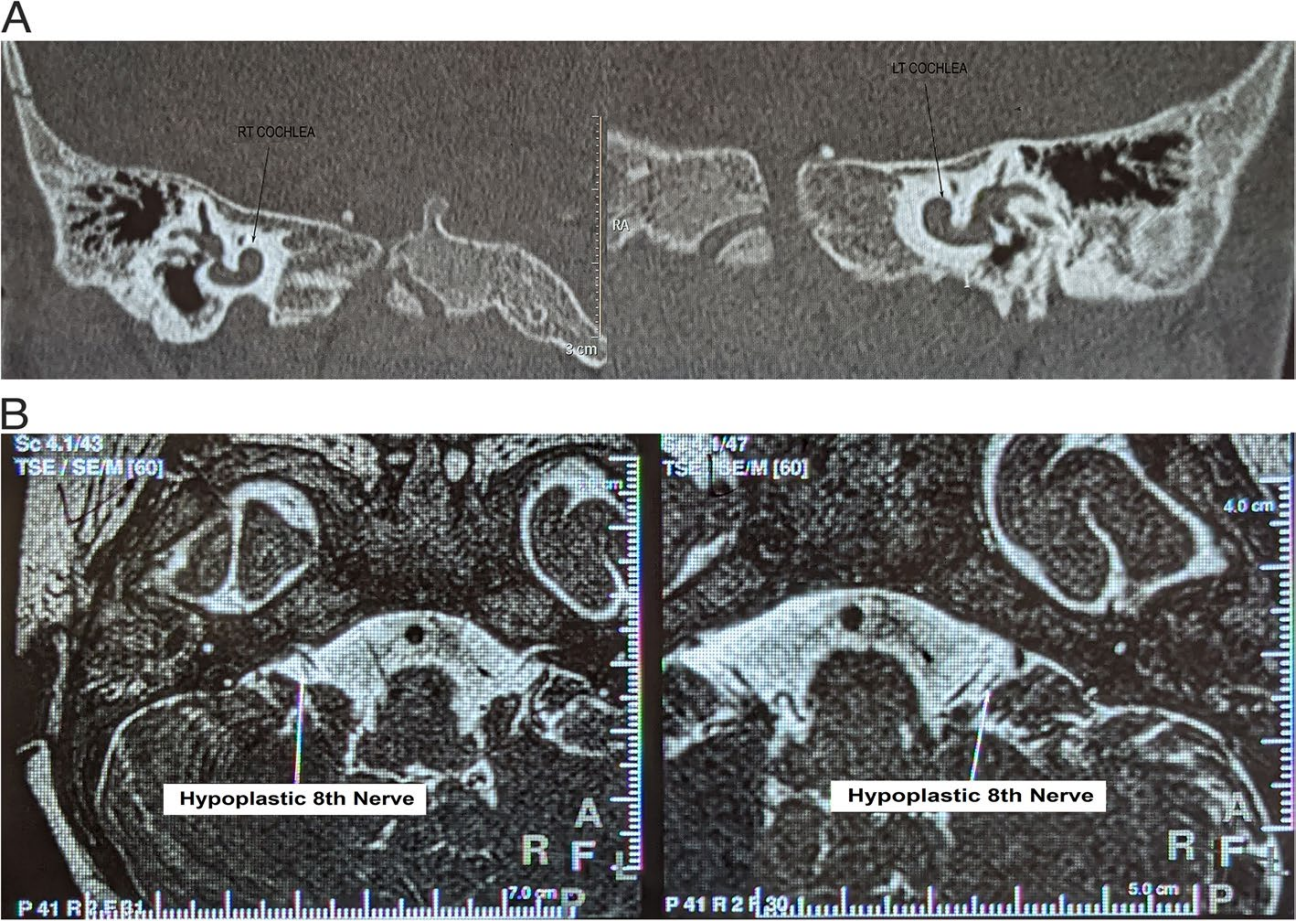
Radiographic images of the inner ear. A) Hypoplastic cochlea with presence of only half basal turns on either side in elder sib. B) Hypoplastic 8^th^ nerves on both sides
